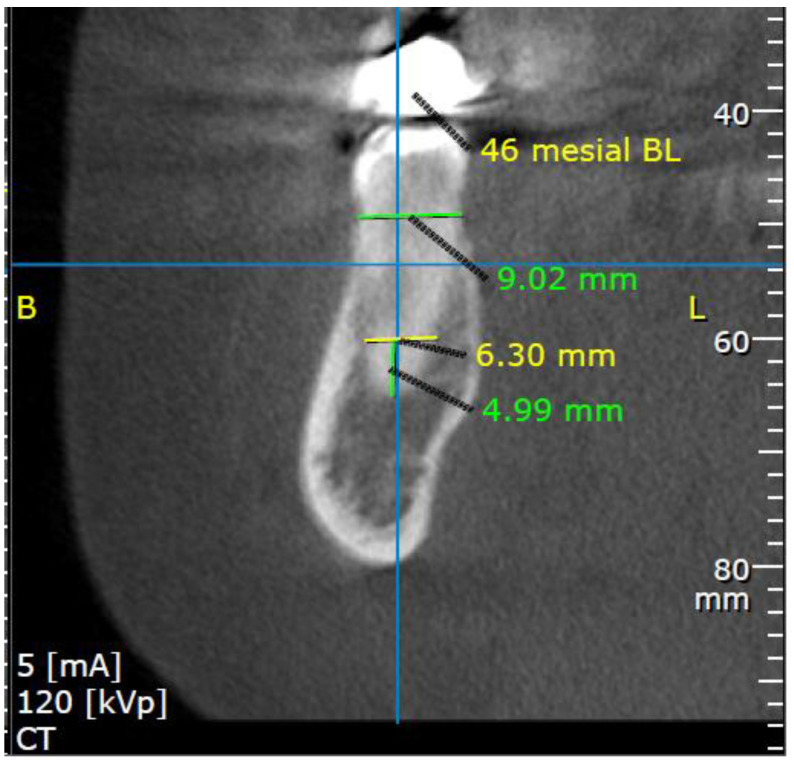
Buccolingual (BL) aspect measurements of right first molar, assessed in 2 different points, referred to tooth long axis: (1) A—5 mm coronal to the apices (4.99 mm from the apex; green line, the BL width is 6.3 mm; yellow line), (2) X- alveolar crest level (BL width is 9.02 mm; green line). CBCT para-axial cross section. B-buccal; L-lingual.
